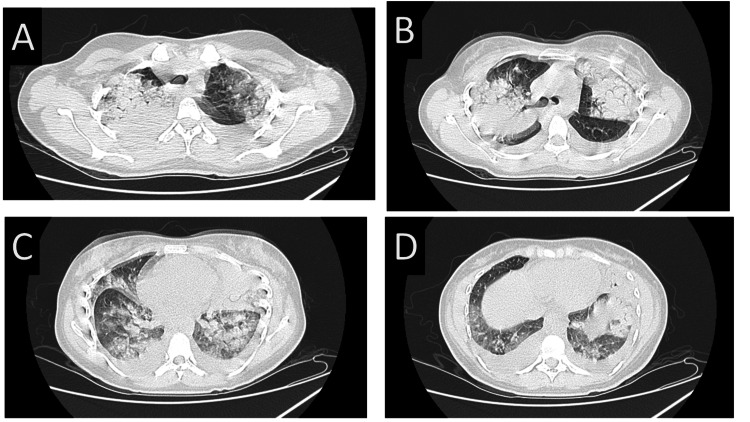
High-resolution CT thorax showing multilobar pneumonia with bilateral consolidations and pleural effusion. (A, B) Bilateral upper lobe consolidation with ground-glass opacities. (C, D) Bilateral lower lobe consolidation, pleural effusion with ground-glass opacities.
